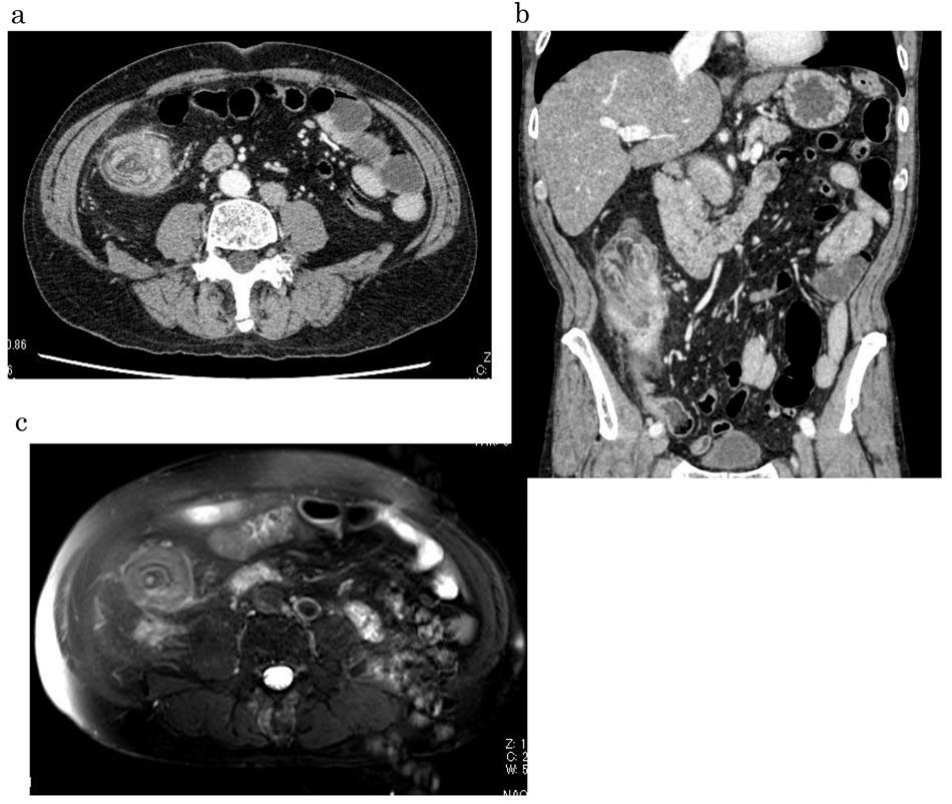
Contrast-enhanced CT/MRI showed increased wall thickness from the ileocecal region to the ascending colon (a, b), which was accompanied by a ring-shaped and layered appearance of the mucosal and muscular layers (c).
